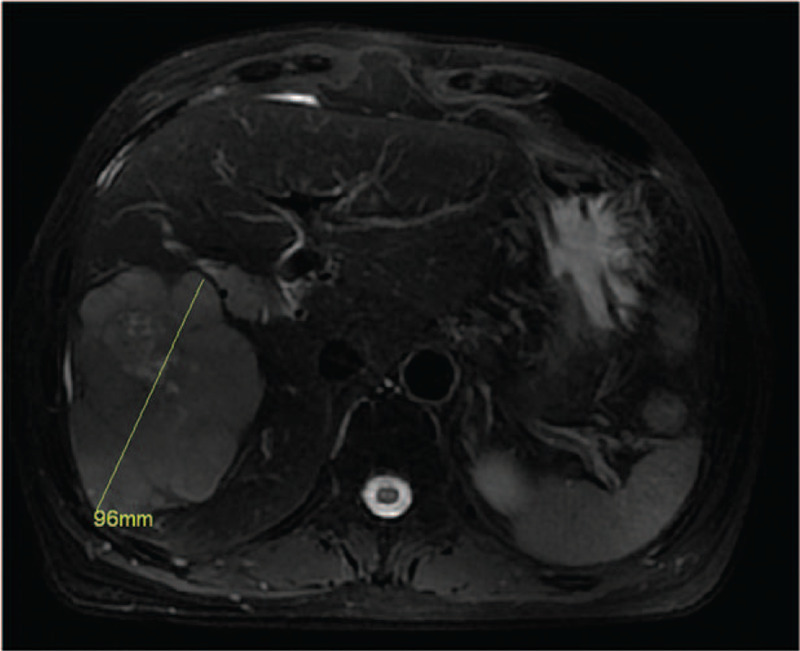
Magnetic resonance imaging scan shows a right huge hepatic mass with portal vein invasion.
